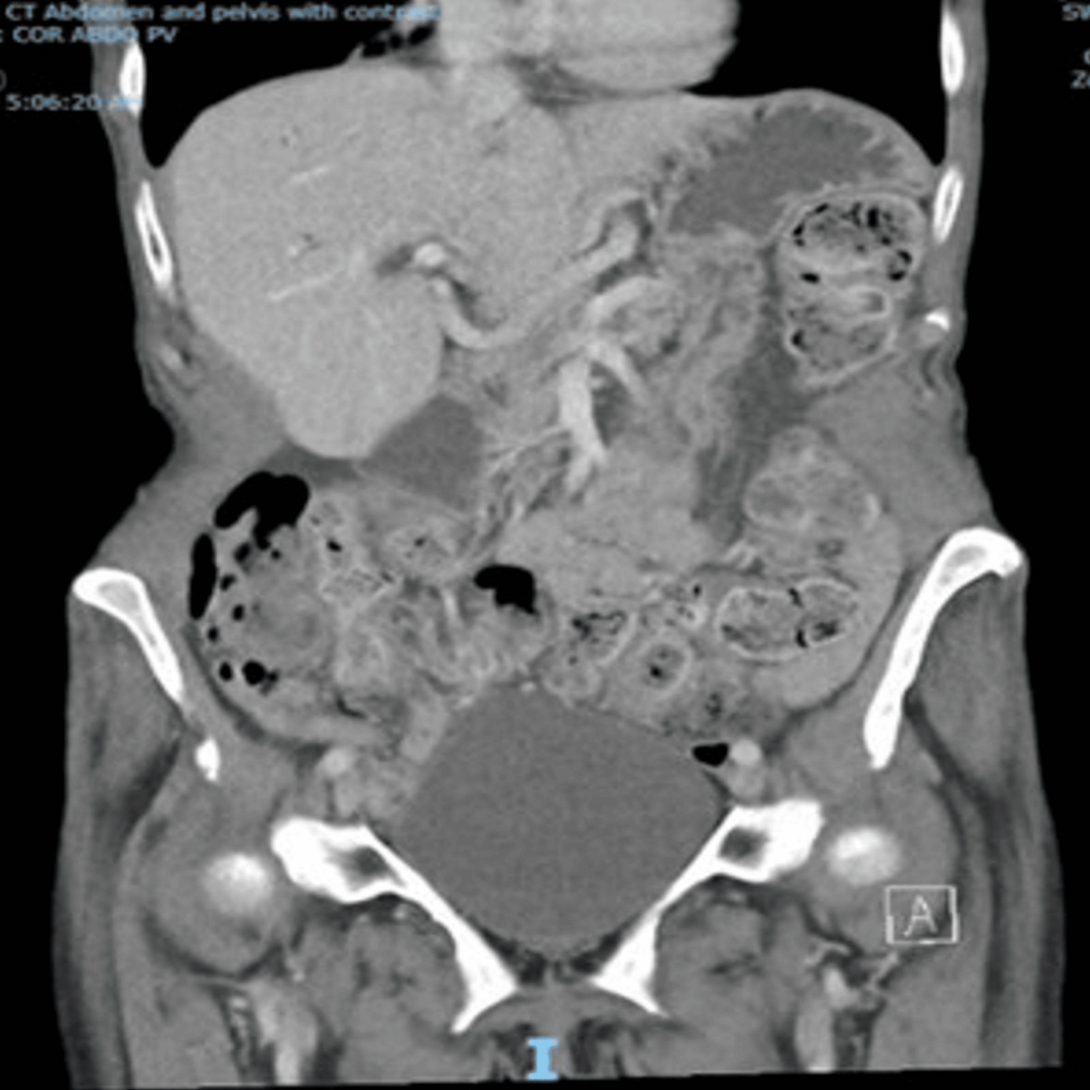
Coronal view of the initial CT revealing mild sigmoid diverticulitis, with no evidence of free fluid or aneurysm CT: computed tomography
